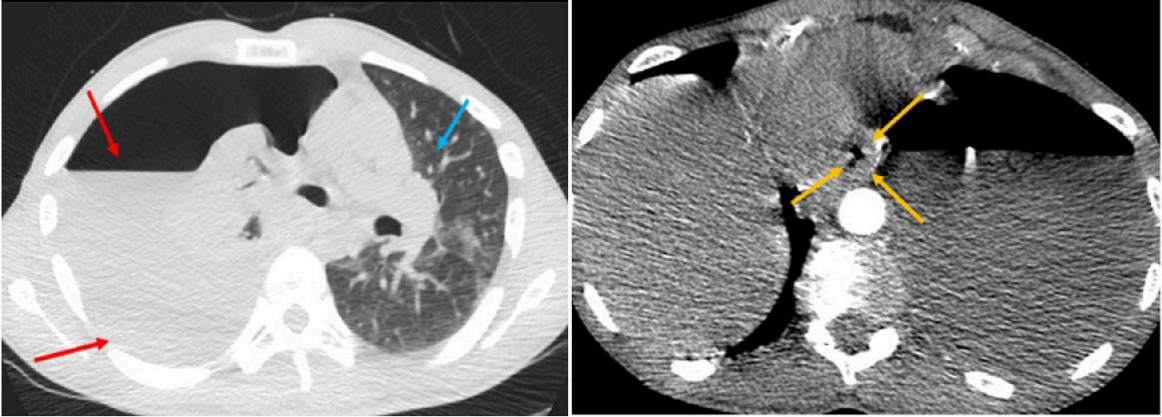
Computed tomography angiogram of the chest (lung and mediastinal window—axial computed tomography). Large left-sided hydropneumothorax (red arrows), oesophageal thickening (yellow arrows), and mediastinal shift to the right (blue arrow).
